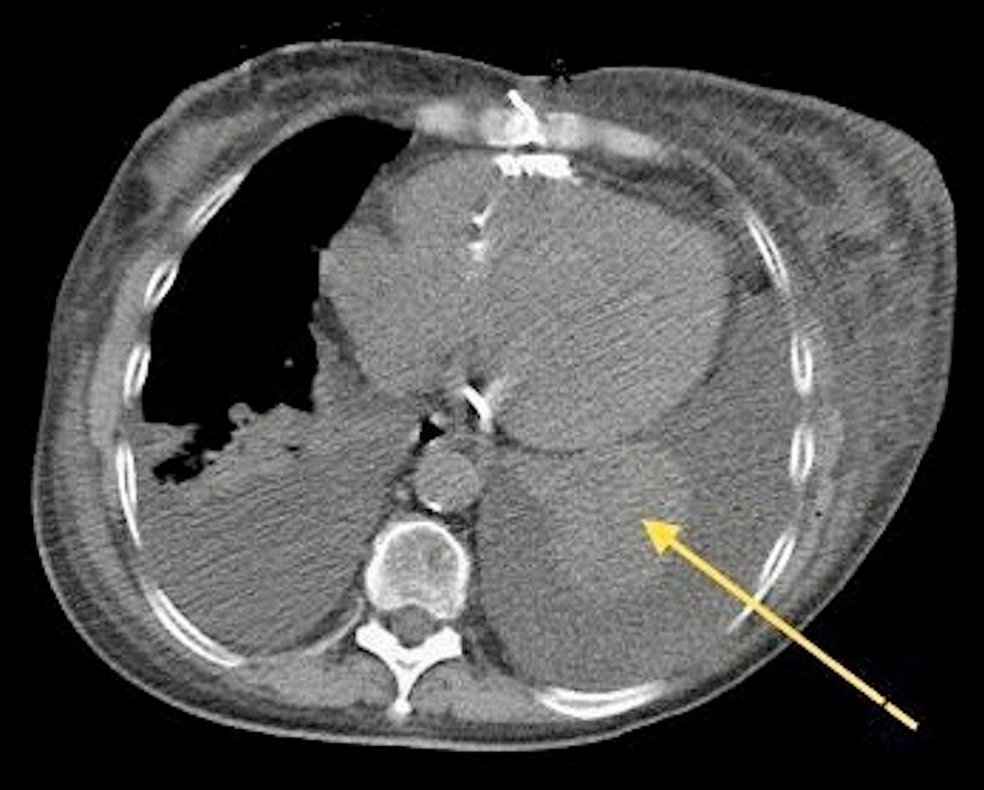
CT thorax showing sub-pleural mass forming an acute angle with the pleura (yellow arrow). CT, computed tomography.
